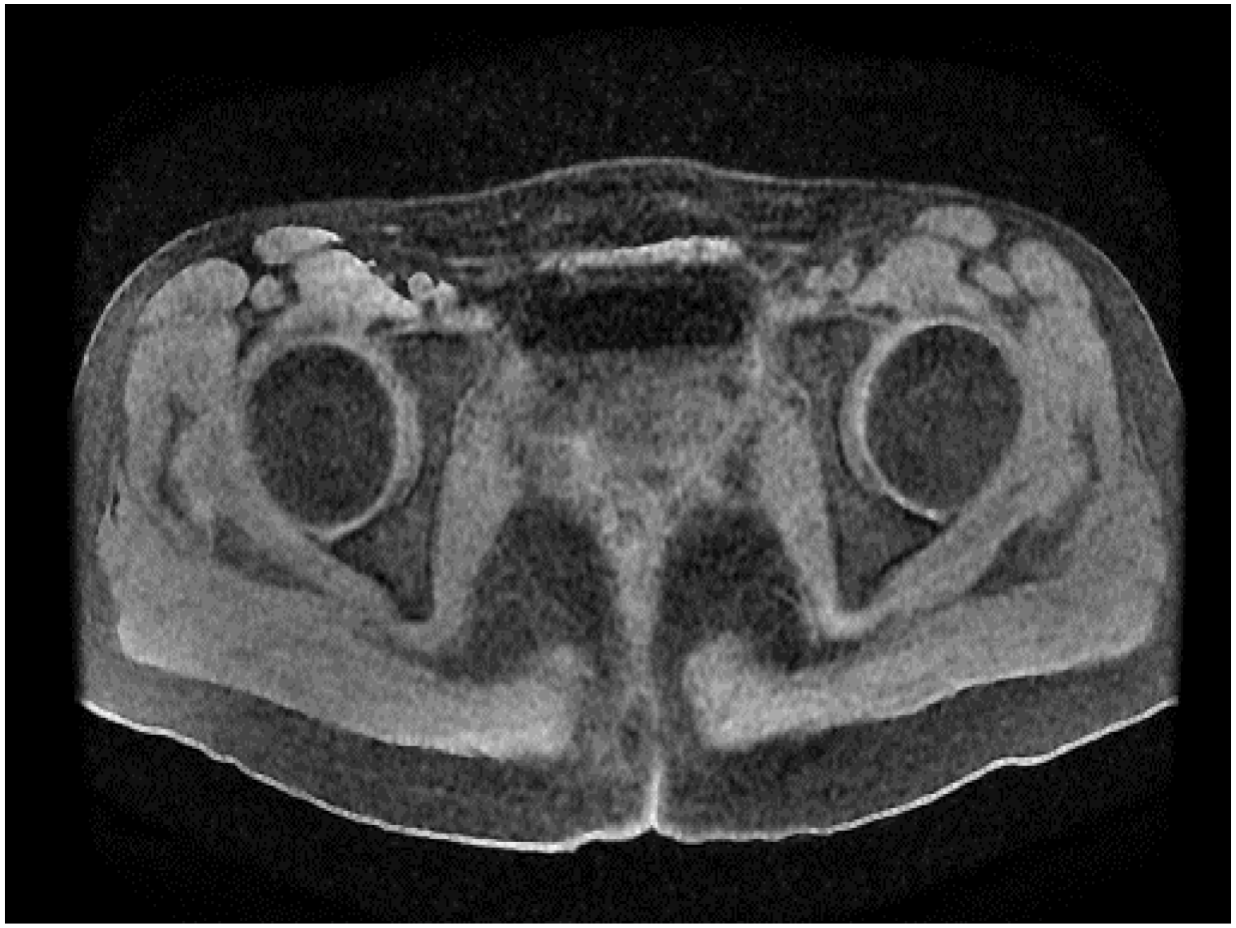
Pelvic MRI findings following six cycles of adjuvant chemotherapy.
